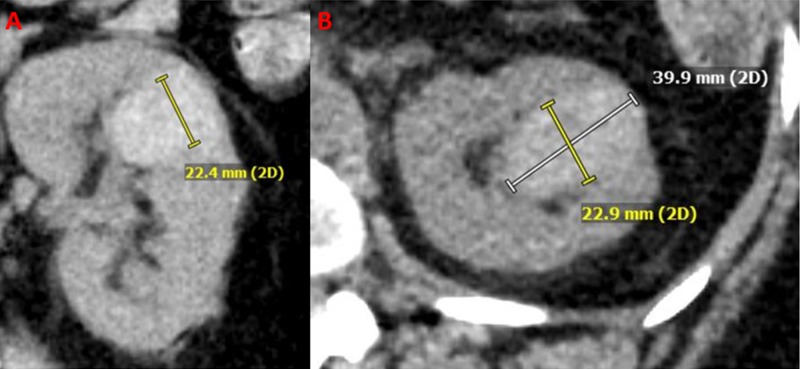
Initial computed tomography (CT). Initial coronal (A) and axial (B) CT images of the renal lesion are shown.
